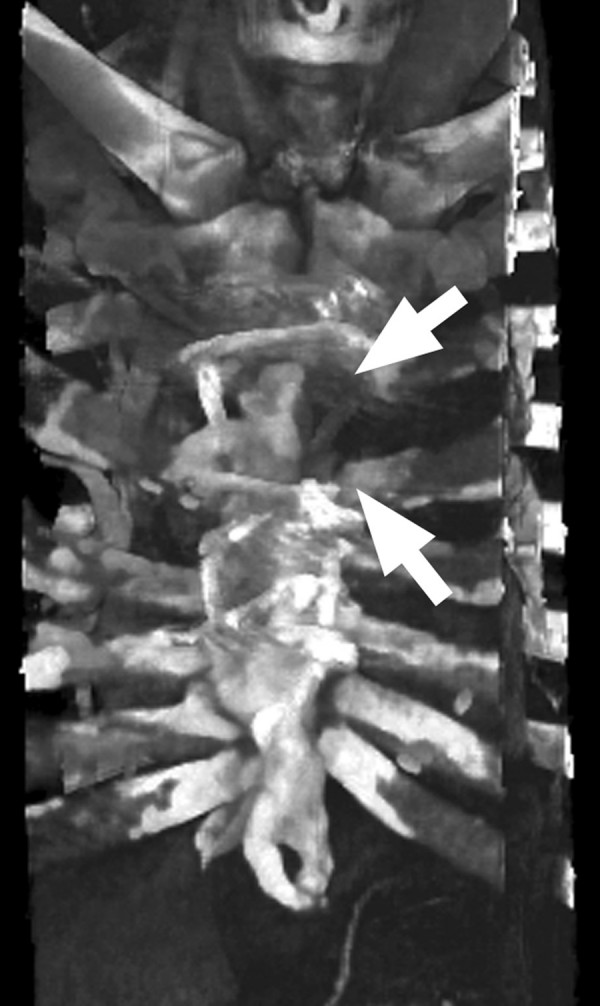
Sternum osteomyelitis. CT 3D-reconstruction shows massive sternal bone defects, inhomogenous bone matrix and irregular sternotomy edges (white arrows) as evidence for long-lasting chronic osteomyelitis
